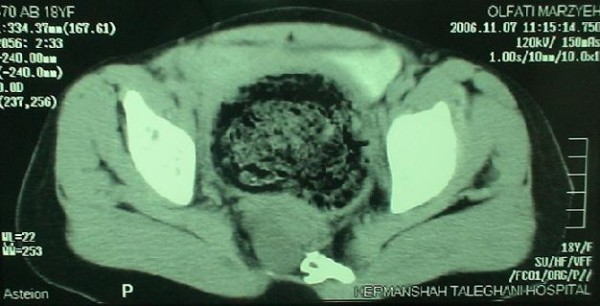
Axial pelvic computed tomography depicting erosion and scalloping of the right hemisacrum by the retrorectal mass.
